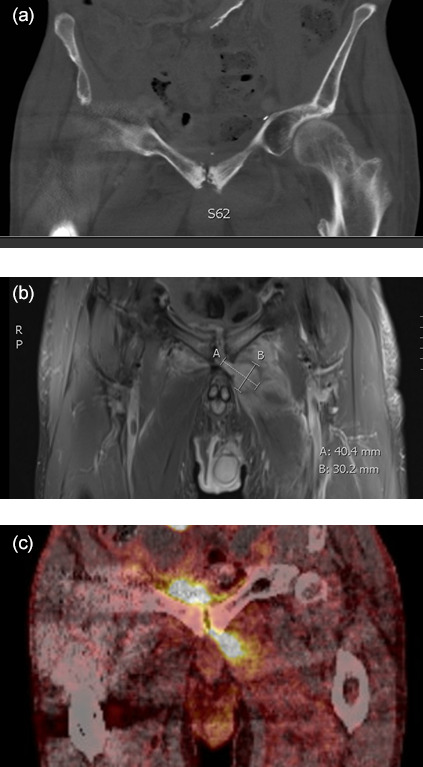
Example of three coronal diagnostic images before surgery from the same patient suggesting SAS diagnosis. (a) CT scan displaying osseous destruction in the symphysis. (b) MRI
